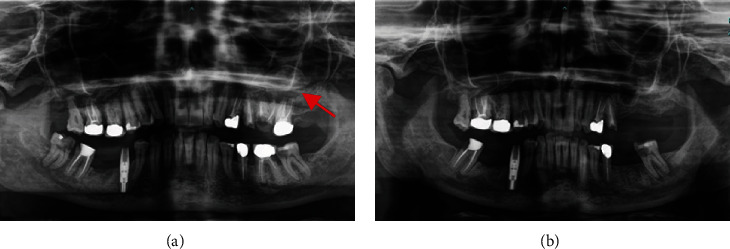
Case 3: (a) preoperative orthopantomography of case 3 showing radiolucent lesion in the left maxilla (red arrow) and (b) postoperative orthopantomography.
